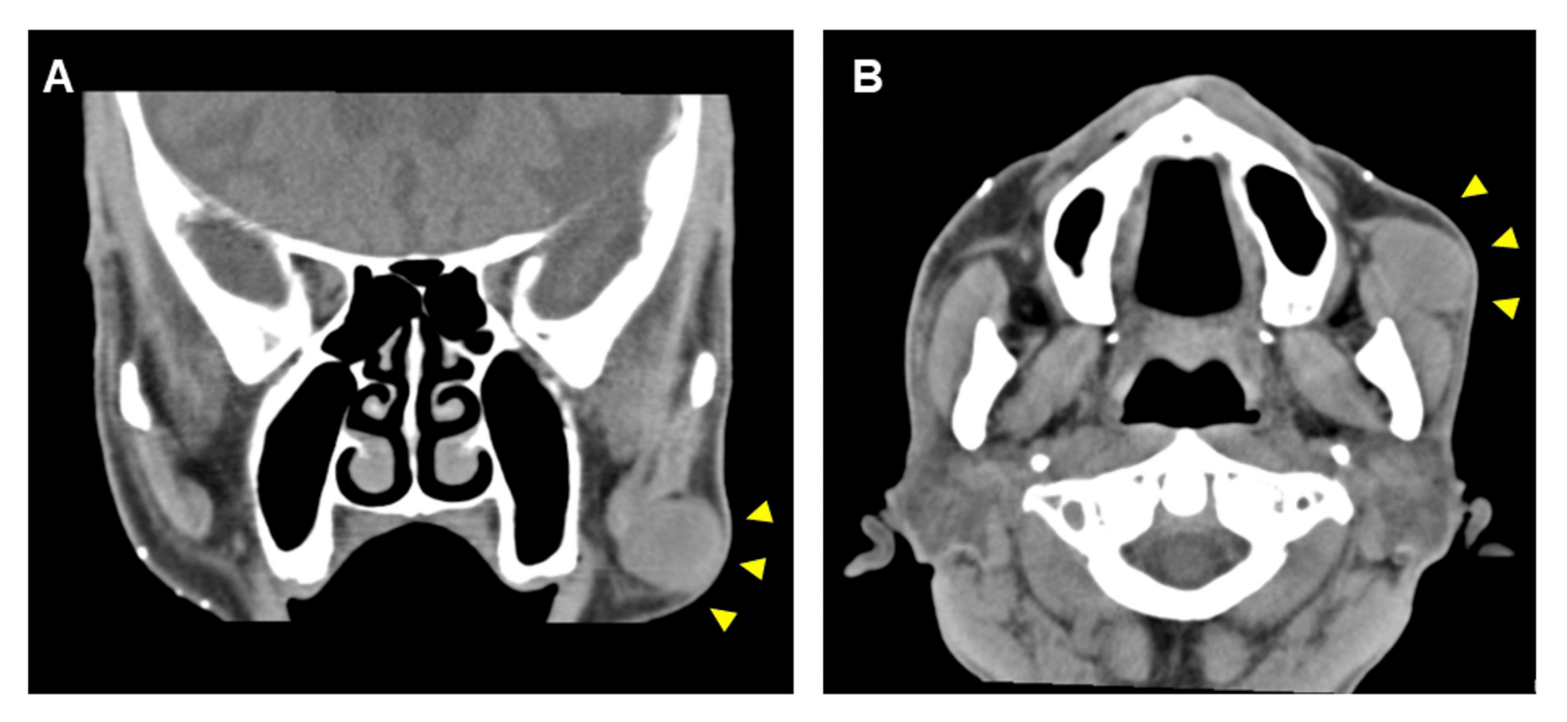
Computed tomography scan of the head and neck. A well-circumscribed iso-dense lesion approximately 30 mm in diameter was observed. A: Coronal section; B: Axial section. Yellow arrowheads indicate the lesion.
